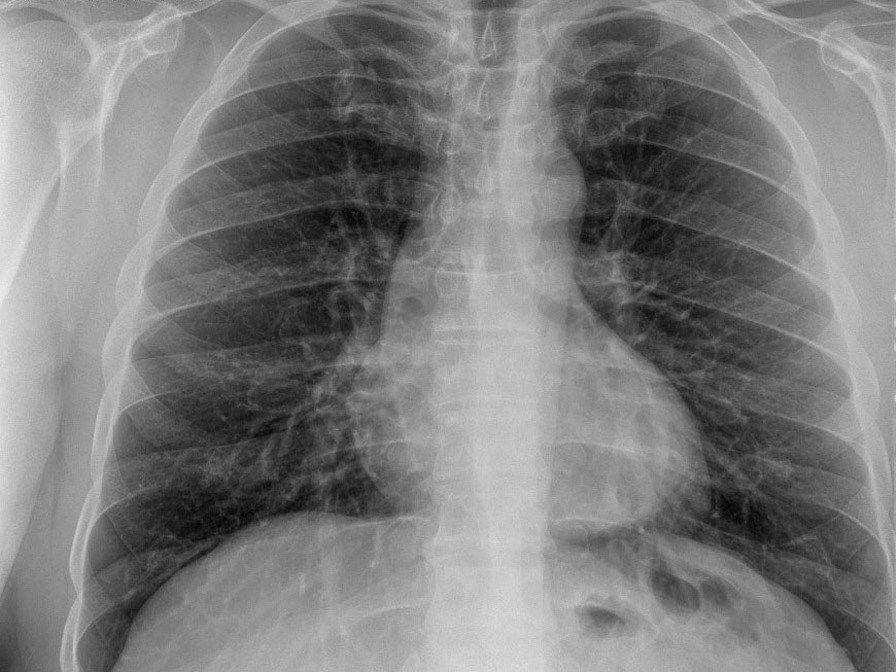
The patient pulmonary X-ray showing a normal appearance at the post-op (18 days after the discharge)
